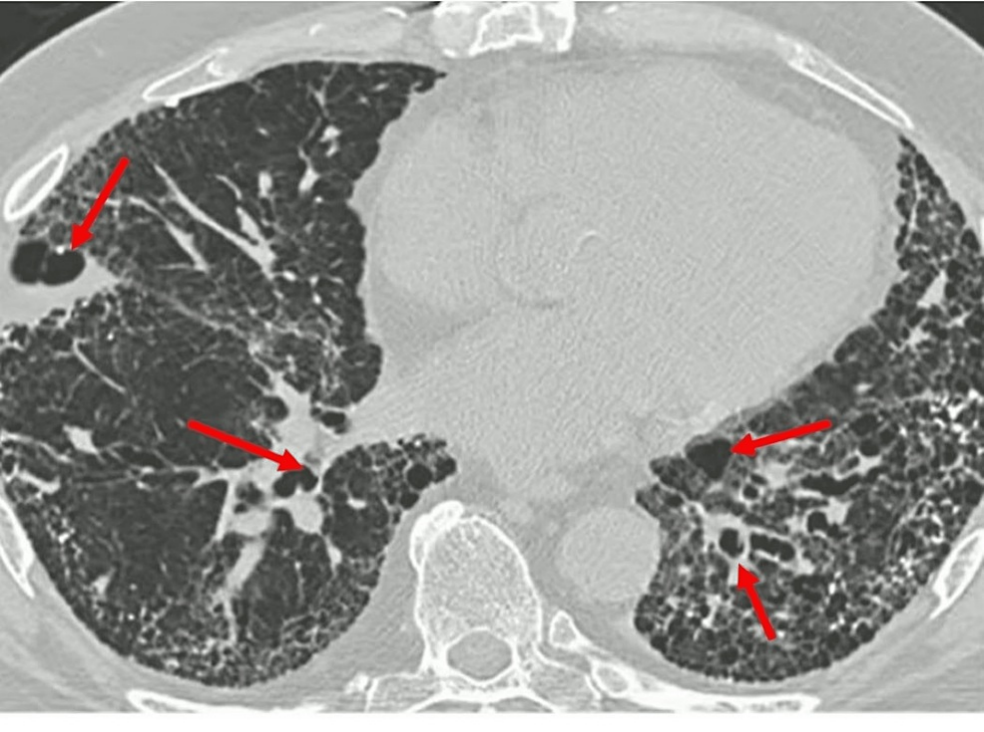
High-resolution CT chest demonstrating ground glass opacities, airway dilation, and thickened airways manifesting bronchiectasis. CT: computed tomography
